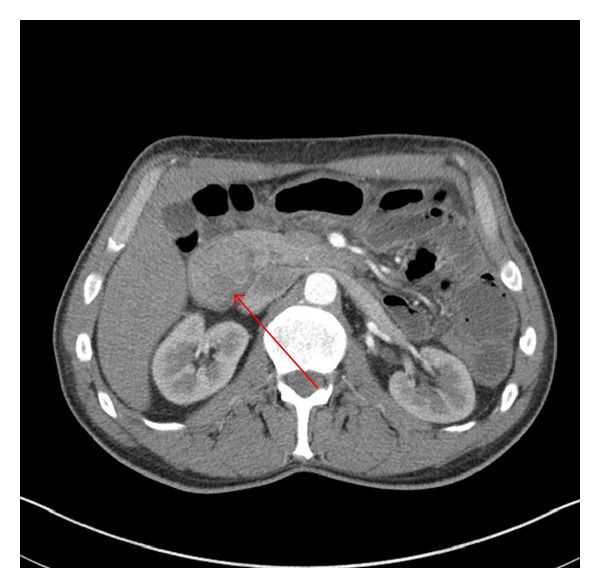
Image from contrast enhanced abdominal CT scan confirming presence of mass lesion in the duodenum (arrow).
